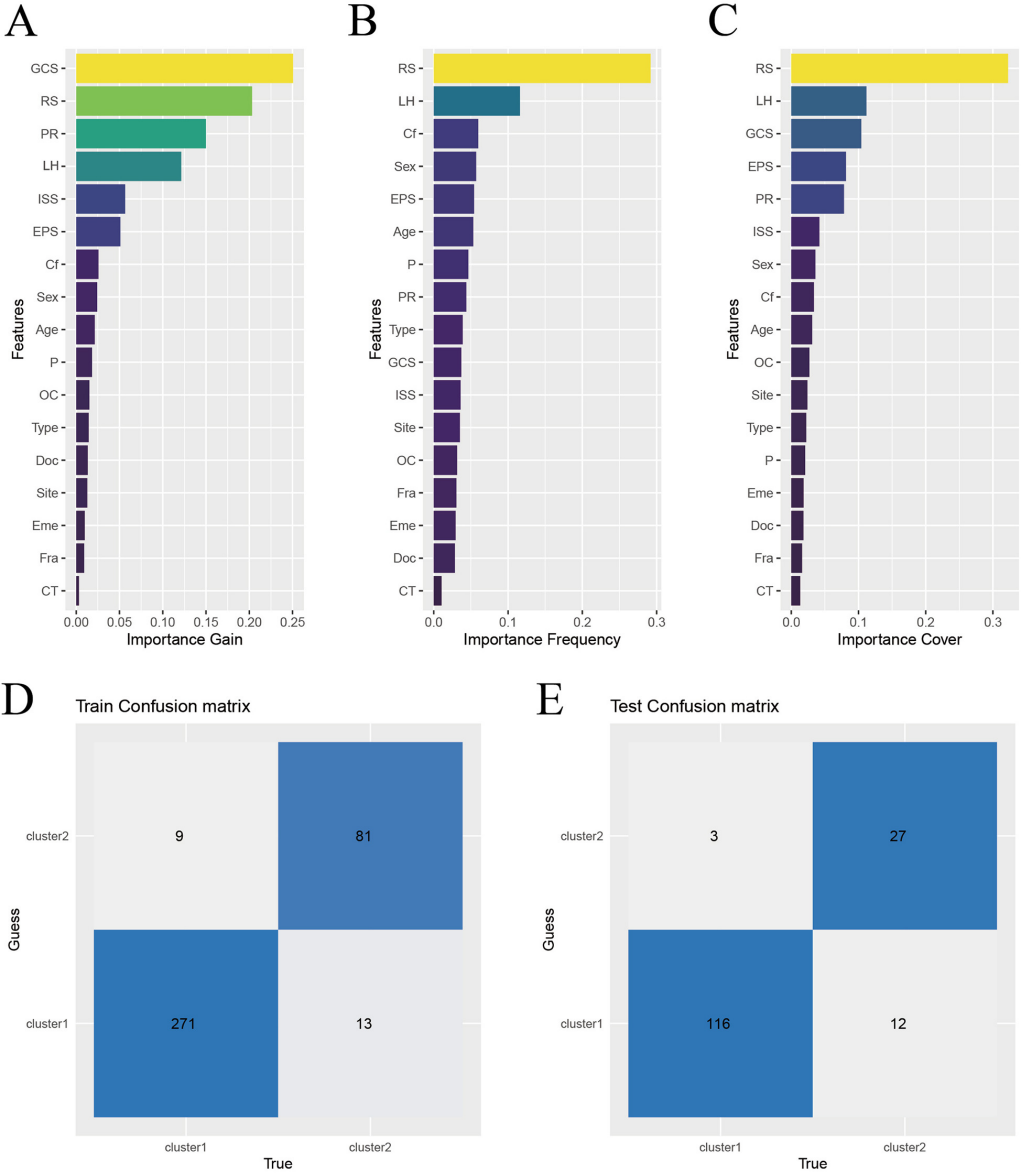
Construction and validation of XGBoost model. (A) Characteristic gain ordering. (B) Ranking of the number of feature splitting points. (C) Sorting of sample number of characteristic sub nodes. (D) Training set confusion matrix. (E) Validation set confusion matrix. Abbreviations: RS, risk score; PR, pupillary light reflex; LH, location of hematoma; EPS, early post-traumatic seizures; Cf, coagulation function; P, injured area (single site or multiple sites); OC, craniocerebral injury (open or close); Type, causes of injury (traffic injury/falling - related injuries/other); Eme: vomiting after injury; Doc, disturbance of consciousness after injury; Site, place of injury (Road/Home/Other); Fra, Skull fracture; CT, Cranial CT.
